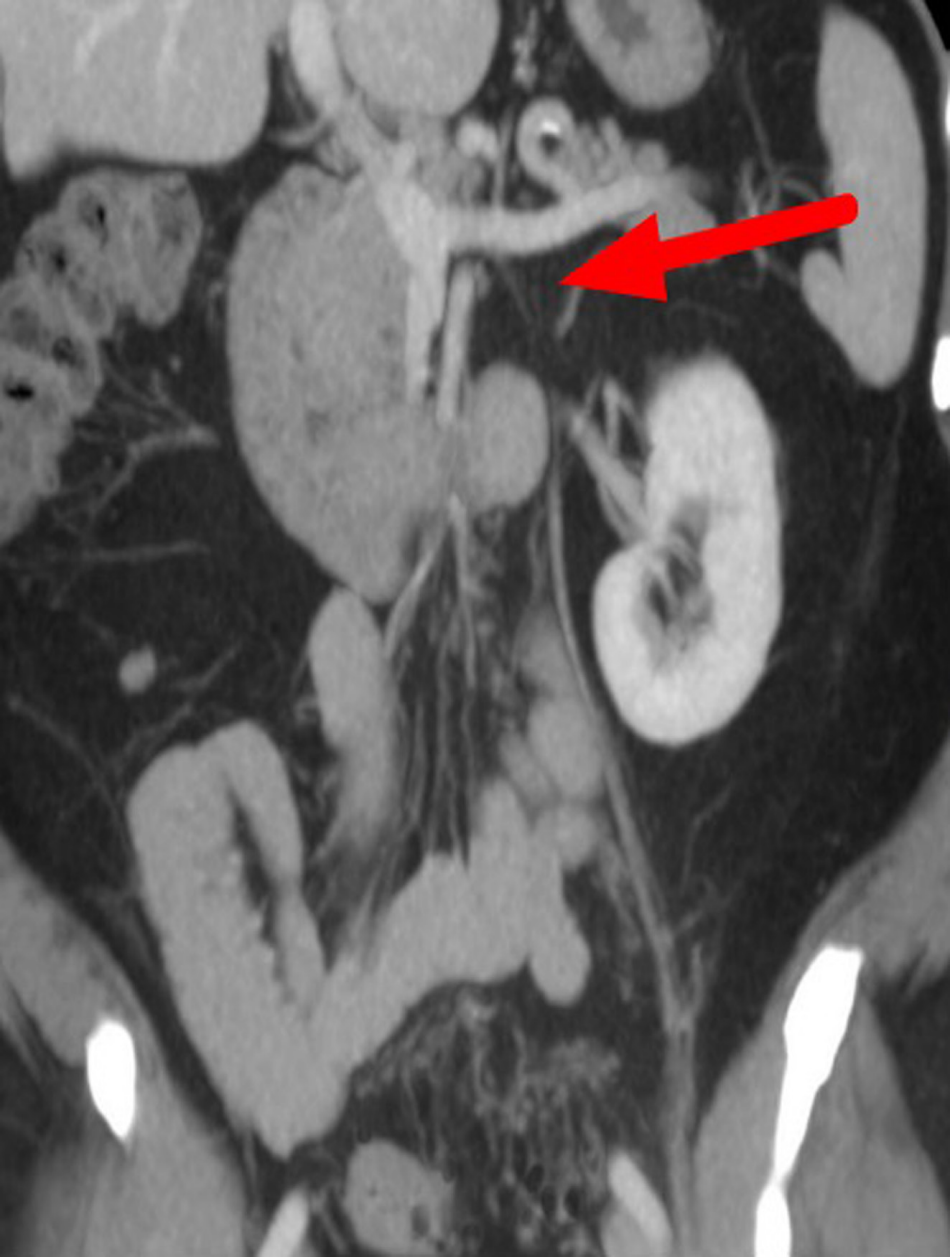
CT abdomen/pelvis 3D coronal view showing atretic appearance of the inferior mesenteric vein compared to the prior study along with resolution of previous perivascular stranding.
